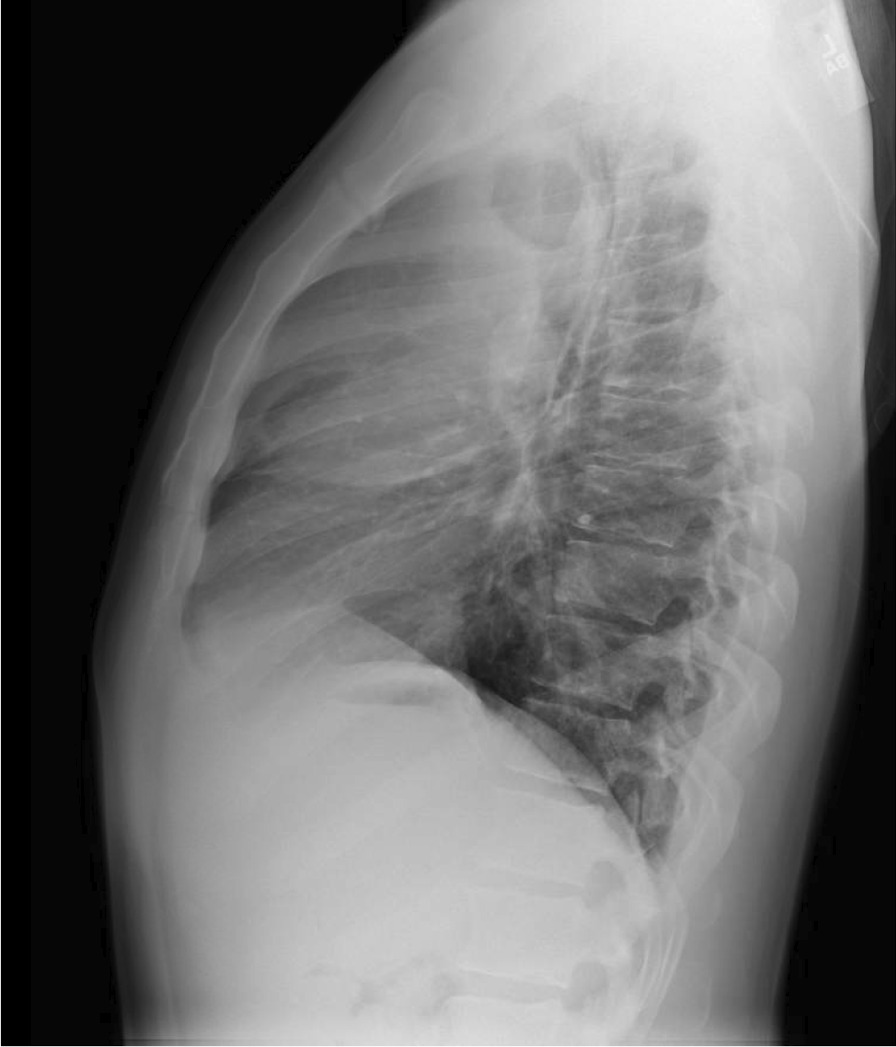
Initial chest X-ray (lateral view). Right upper lobe cavitary lesion
